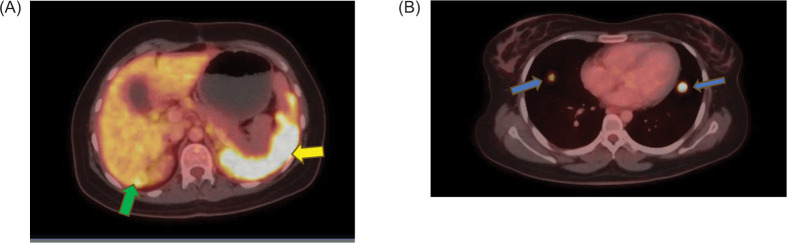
(A) PET CT scan in in August 2025 after 1st line chemotherapy showed recurrent lesions (Yellow Arrow) in the left subdiaphragmatic and peri-splenic regions, along with liver metastases (Green Arrow) and bilateral lung metastasis (Blue Arrow).
